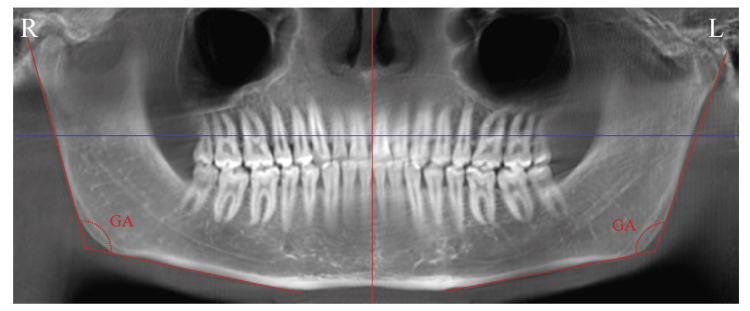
Measurement of the gonial angle on CBCT images CBCT: Cone-beam computed tomography; GA: Gonial angle; R: Right; L: Left.
